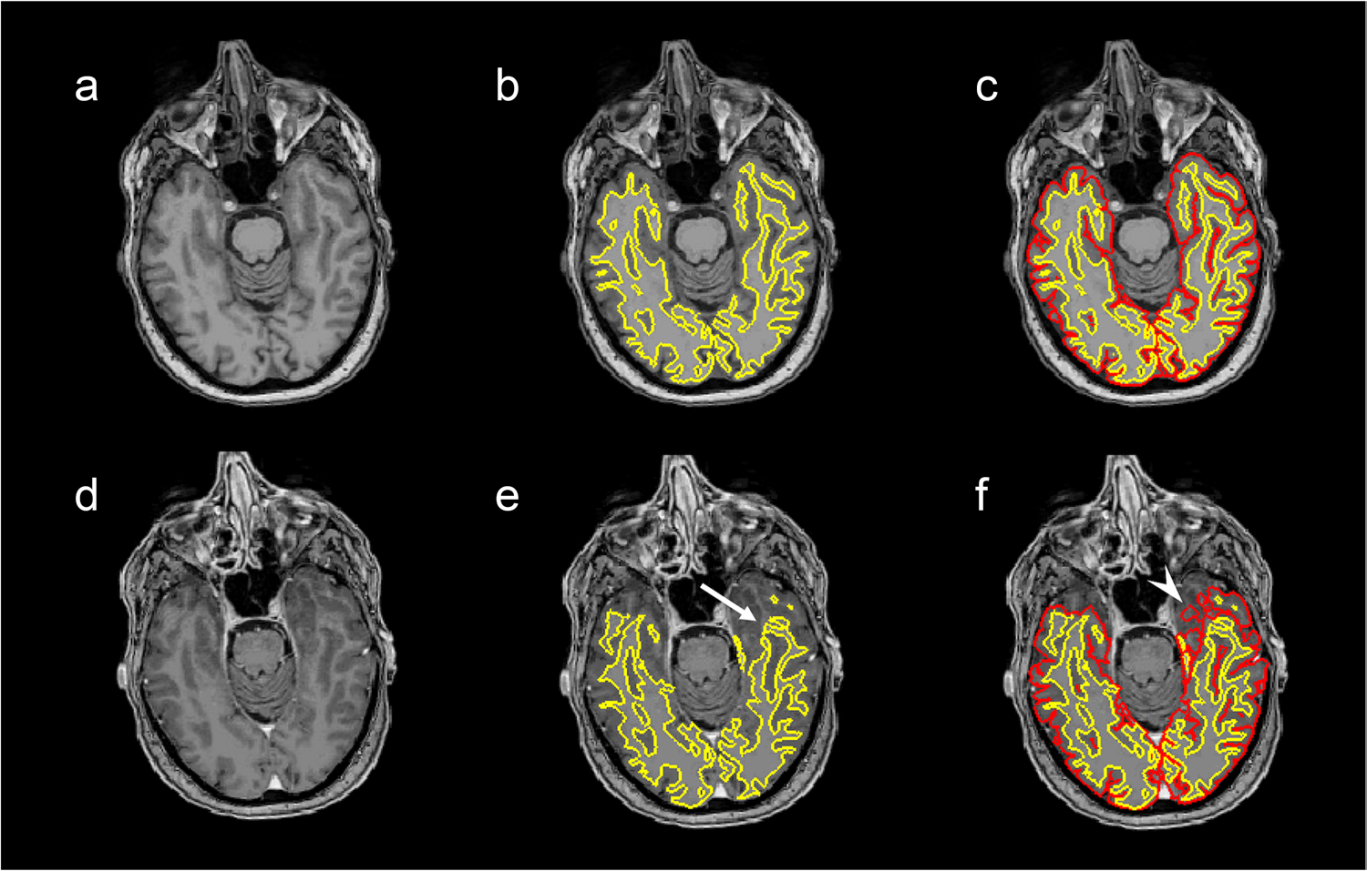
Pre-contrast (a–c) and post-contrast (d–f) T1-weighted images obtained from the same patient (subject A3) in the same MRI session. b and e show the white surface, which is the border between white and grey matter as automatically constructed by FreeSurfer (yellow). c and f show the pial surface, which is similarly the automatically constructed border between grey matter and cerebrospinal fluid (red), derived from the white surface. The figure demonstrates a typical failure of moderate degree, where the white surface fails to include parts of the temporal poles in the post-contrast image (e) (arrow), with subsequent mistakes in the pial surface (f) (arrowhead)
